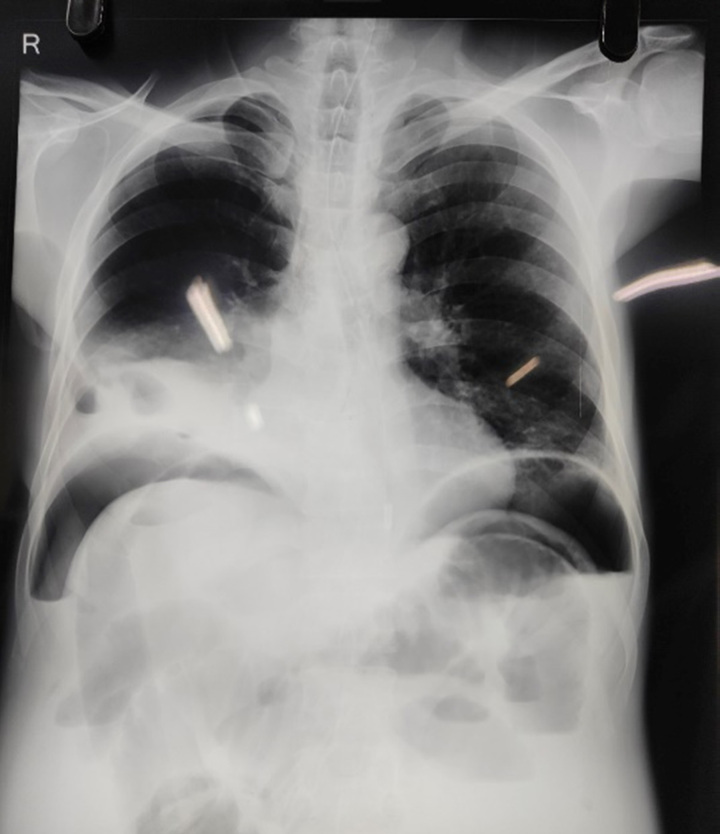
Preoperative chest radiograph showing radio-opaque area over the lower one-third of right lung suggestive of bowel herniation.
